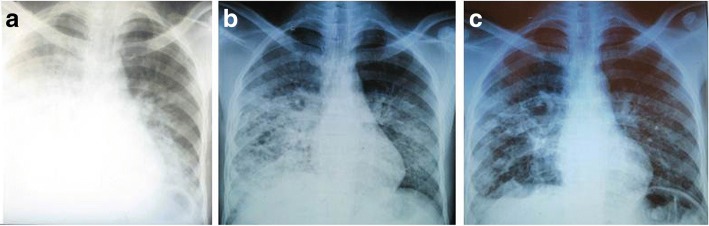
Chest X-ray of the pneumonic plague patient. a on admission at Miarinarivo District Hospital: chest X-ray image revealed right pleural effusion and radio-opacities of left lower lobe, (b) On presentation to the Military Hospital of Antananarivo (10 days after A): enlargement of left opacities and appearance of right nodular opacity, decrease of right pleural effusion, and (c) Upon discharge at Anti-plague Hospital of Ambohimiandra- Antananarivo (9 days after B), decrease of right opacity and left pleural effusion
